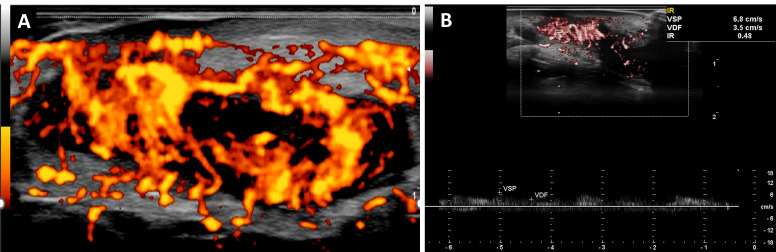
(A) High-resolution Power Doppler ultrasound (12.5 MHz; PRF 480 Hz) demonstrates a hypoechoic dermo-subcutaneous inflammatory complex corresponding to an abscess cavity and associated tunnel formation. Doppler settings were optimized for low-velocity flow detection, revealing dense intralesional and perilesional vascular signals. The marked hypervascularization reflects active inflammatory angiogenesis and microvascular remodeling, consistent with validated Doppler-based inflammatory biomarkers in HS [2,3,4,5]. (B) Spectral Doppler interrogation of an intralesional vessel demonstrates a low-resistance arterial waveform with a resistive index (RI) of 0.48, supporting active inflammatory hyperemia and neovascular activity. The low RI pattern is consistent with inflammatory-driven vascular proliferation rather than fibrotic or quiescent tissue. These findings reinforce the role of Doppler ultrasound as a noninvasive biomarker of disease activity and vascular dynamics in HS [6]. The same Doppler acquisition parameters were applied for the Doppler images shown in Figure 4 and Figure 6.
